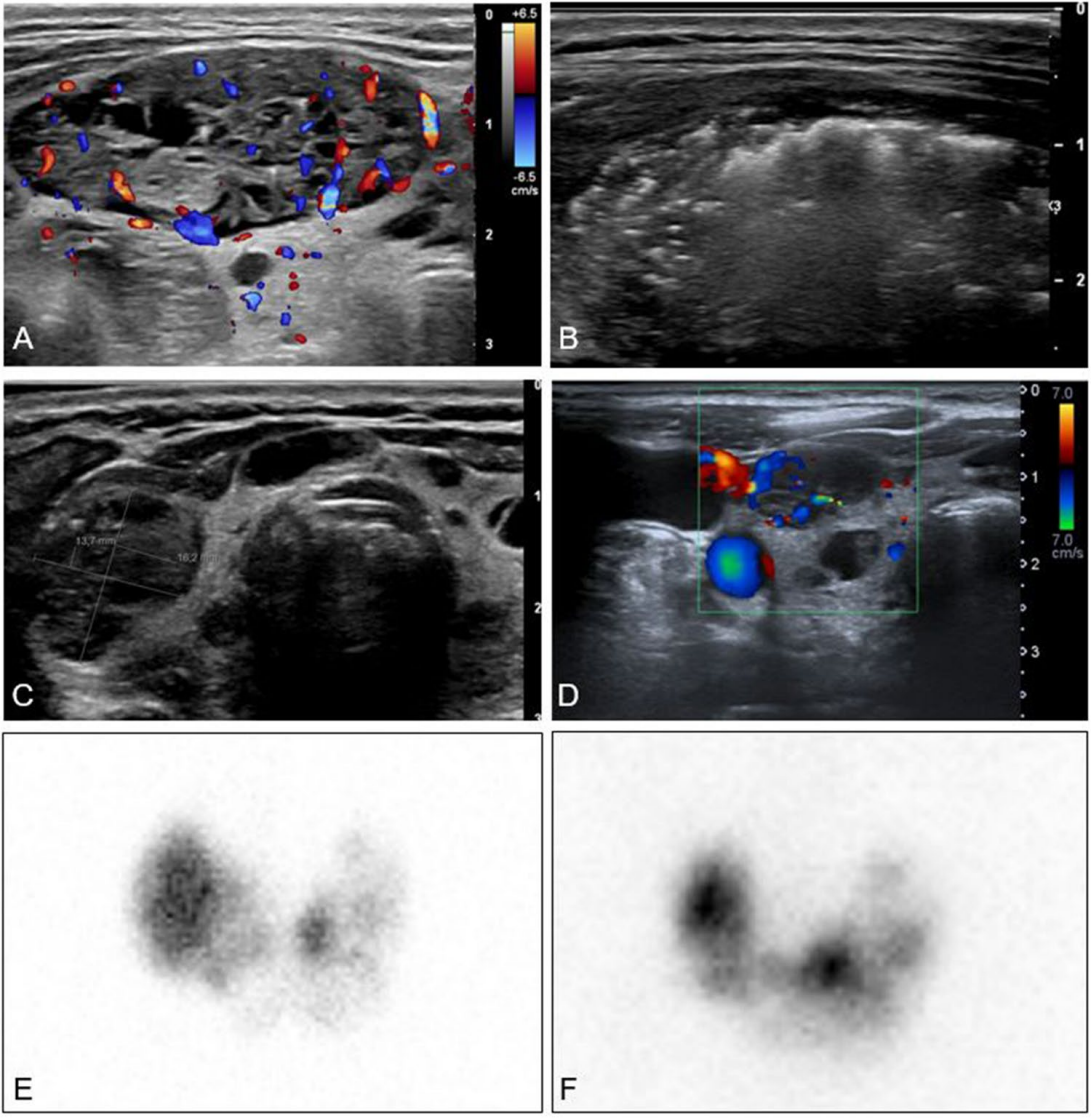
A–D Colour Doppler and grey scale ultrasound images of a patient successfully treated with RFA for TMG. A Before RFA, showing a hypervascular nodule, volume 8.9 mL. B During RFA procedure with typical hyperechoic zones. C 4 months after RFA, volume 2 mL. TSH has increased from below assay limit at baseline to 0.24 mU/L. D 1 year after RFA treatment, volume 1.1 mL. Reduction of vascularity of nodule but some vascularity in periphery of nodule. 2E-F Image of thyroid scintigraph using 123I as tracer. E Before RFA depicting inhomogeneous uptake with a right sided hot nodule. F 17 months after RFA. Reduction of volume and autonomy of right sided nodule and persistent look of inhomogeneous uptake
